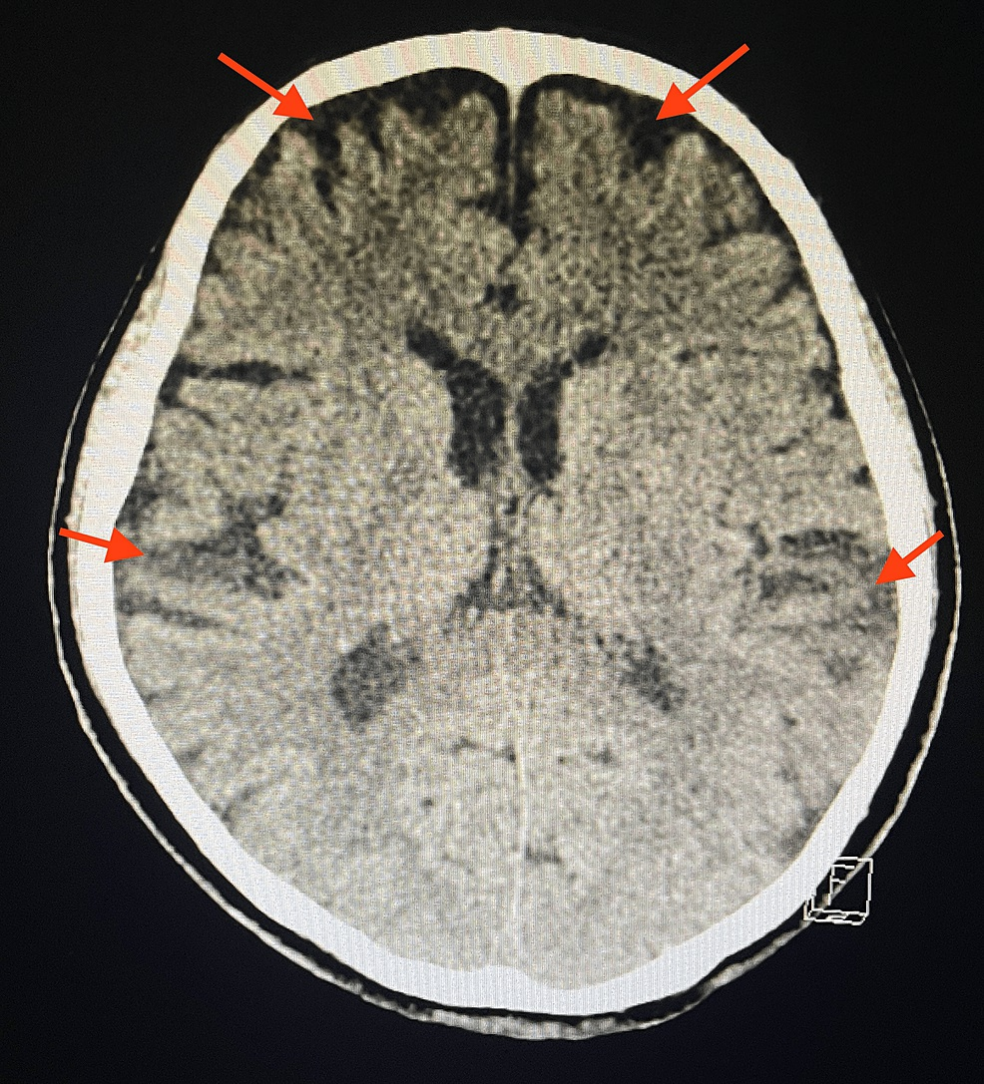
Non-contrast CT brain imaging performed in the emergency department. Non-contrast CT brain imaging showed that the ventricles, sulci, and cisterns were mildly prominent due to diffuse mild atrophy (red arrows). No midline shift, mass effect, mass lesion, hemorrhage, extra-axial collections, and acute infarction or fluid collections were seen.
